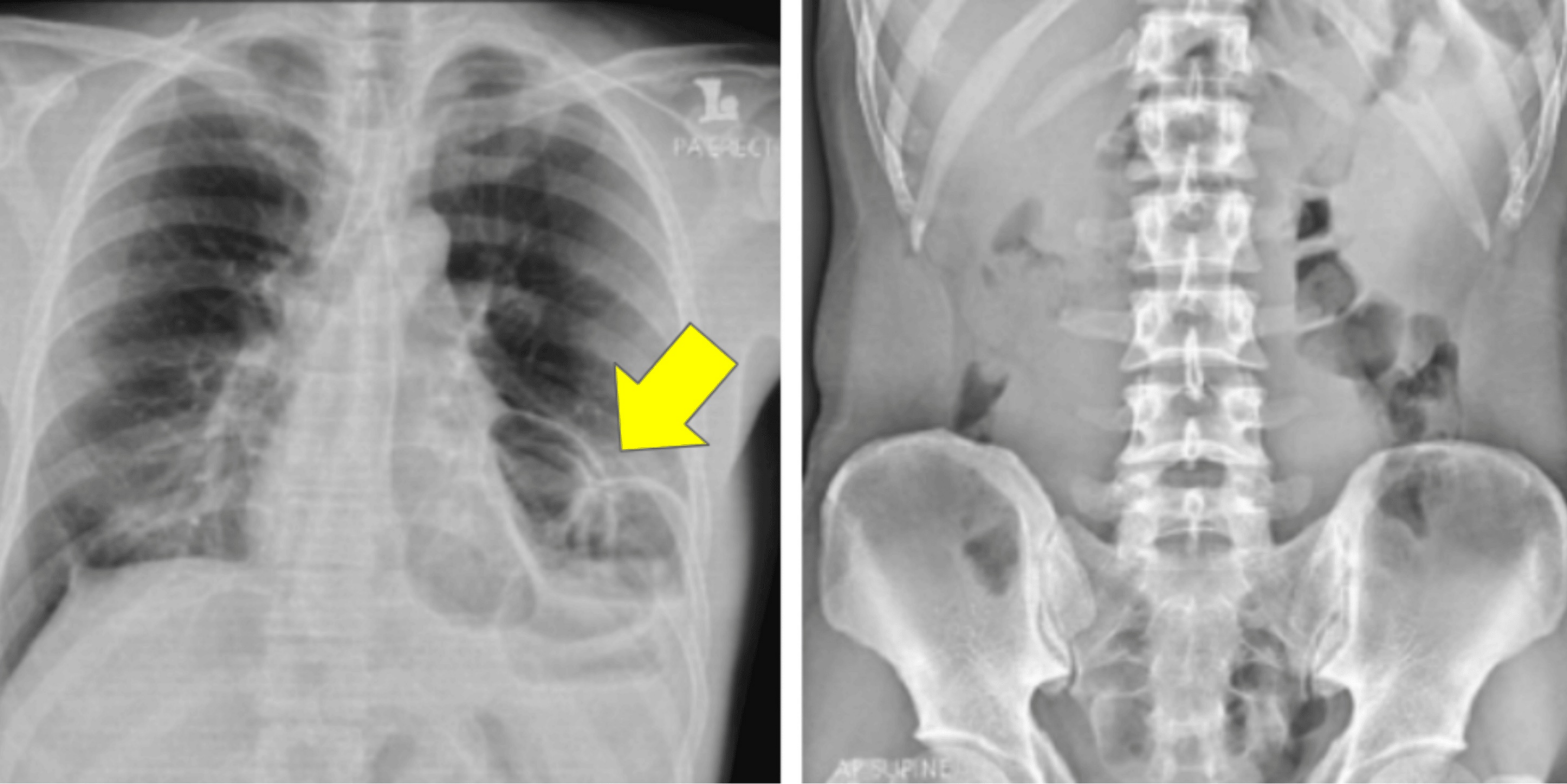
Chest and abdominal radiograph during acute presentation Chest X-ray noted bowel loop at the left hemidiaphragm (yellow arrow). No dilated bowel in the abdominal X-ray.
